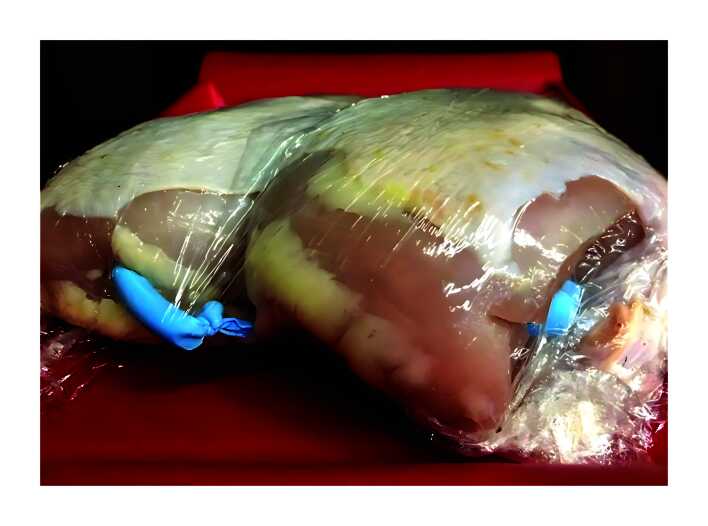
Puncture model comprising chicken breast and tubular latex balloon filled with ultrasound gel. Source: authors’ files.
